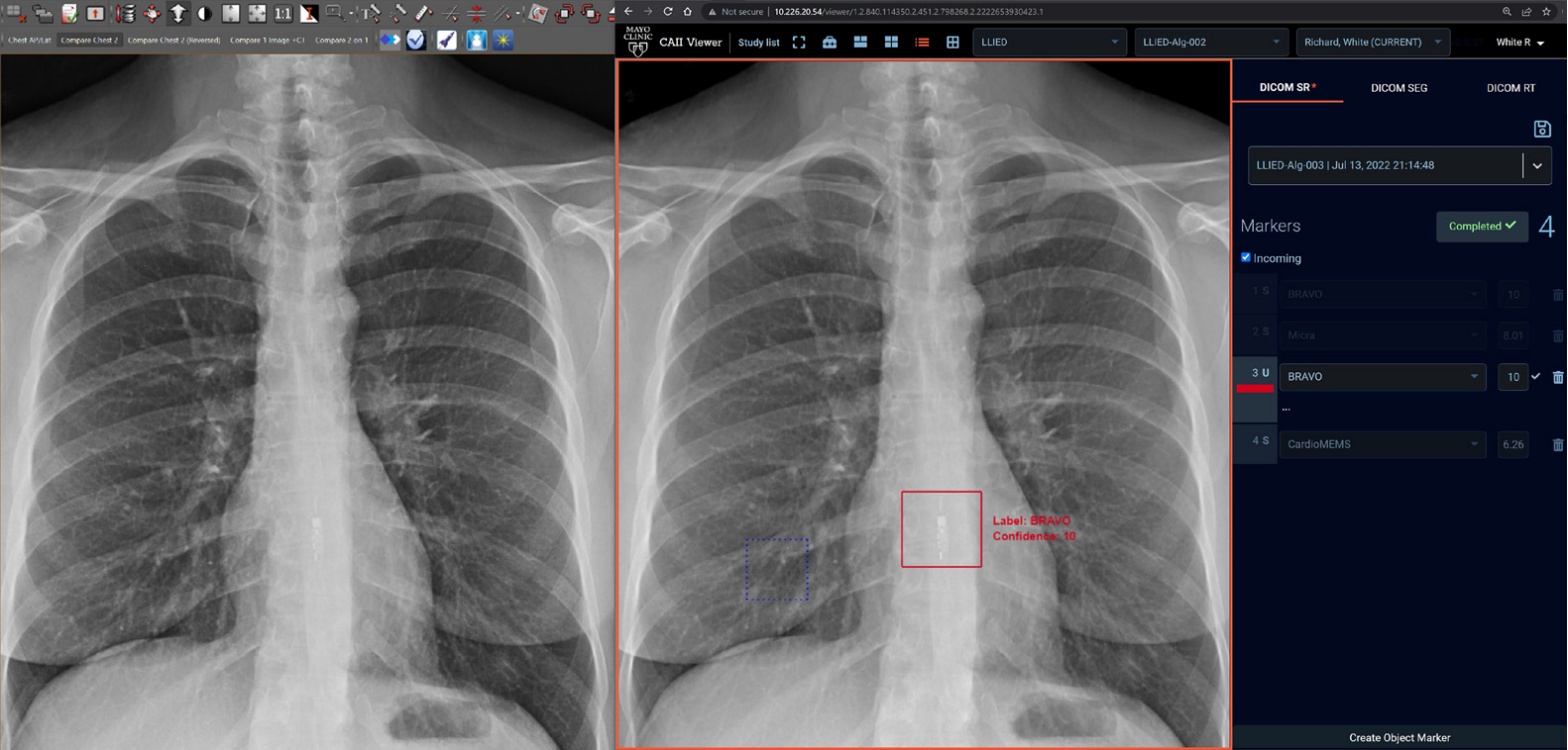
Simultaneous adjacent clinical PACS and ZF GUI/viewer displays. In the limited-deployment test environment, the standard clinical PACS display (a) is simultaneously accompanied by an adjacent display of inference results by the ZF GUI/viewer (b) on the same monitor used by radiologist for routine clinical image interpretations, although not yet integrated. On this frontal CXR image, the 12-class updated LLIED model has correctly detected/localized and identified (with GBB) the MRI-unsafe ERC (Bravo™ Reflux Capsule), with immediate single-click positive adjudication (Red boundaries applied with acceptance) versus passive rejection of any false-positive GBBs (maintained blue boundaries).
